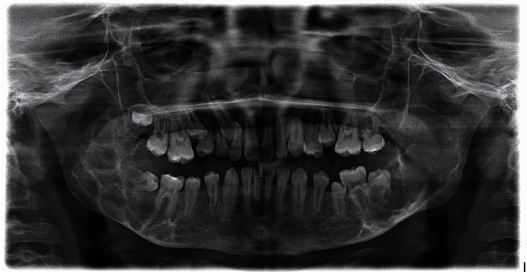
Orthopantomography demonstrating bilateral poorly defined multilocular radiolucent areas in the mandible involving the posterior body, angle, and coronoid process. There was impaction of several teeth in the maxilla and the second molars in the lower jaw. There was root resorption of the tooth #18, 19, and 31.
